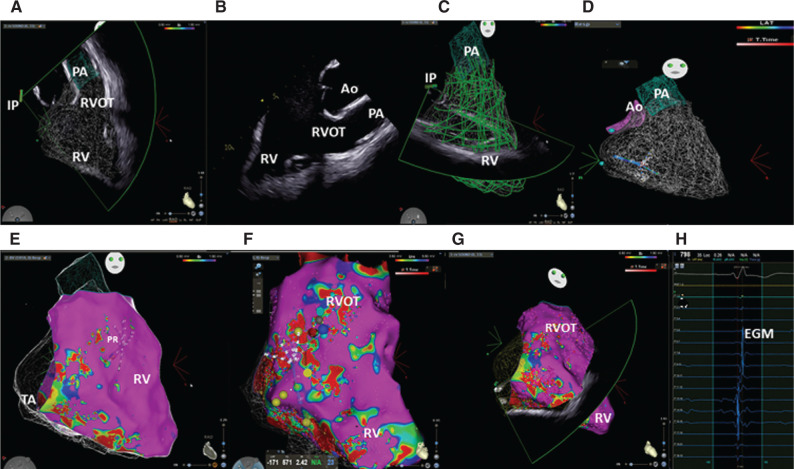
A: In the 3-dimensional intracardiac echocardiography (ICE) map, the probe is positioned in the right atrium; this is the ICE home view. B: Two-dimensional (2D) ICE visualization of the inner and outer tracts of the right ventricle (RV); this is the 2D home view visualization. C: 2D ICE visualization of the RV in the transversal view. The green segments are the beams acquired to obtain a 3D echocardiographic RV map. D: 3D ICE map of the RV. A multipolar catheter is in the inner tract of the RV. E: A merged ICE and bipolar voltage map is represented. Low-voltage areas were stored at the sub-tricuspidalic annulus and at the inferior apex of the RV. F: A merged ICE and voltage unipolar map is represented. It shows the low-voltage areas in the anteroseptal outer tract. G: A merged ICE and voltage bipolar map; real-time ICE probe beam of the RV during voltage mapping. H: Low potential stored. *Abbreviations:* Ao, aorta; IP, ice probe; PA, pulmonary artery; RA, right atrium; RVOT, right ventricular outer tract; TA, tricuspid annulus.
